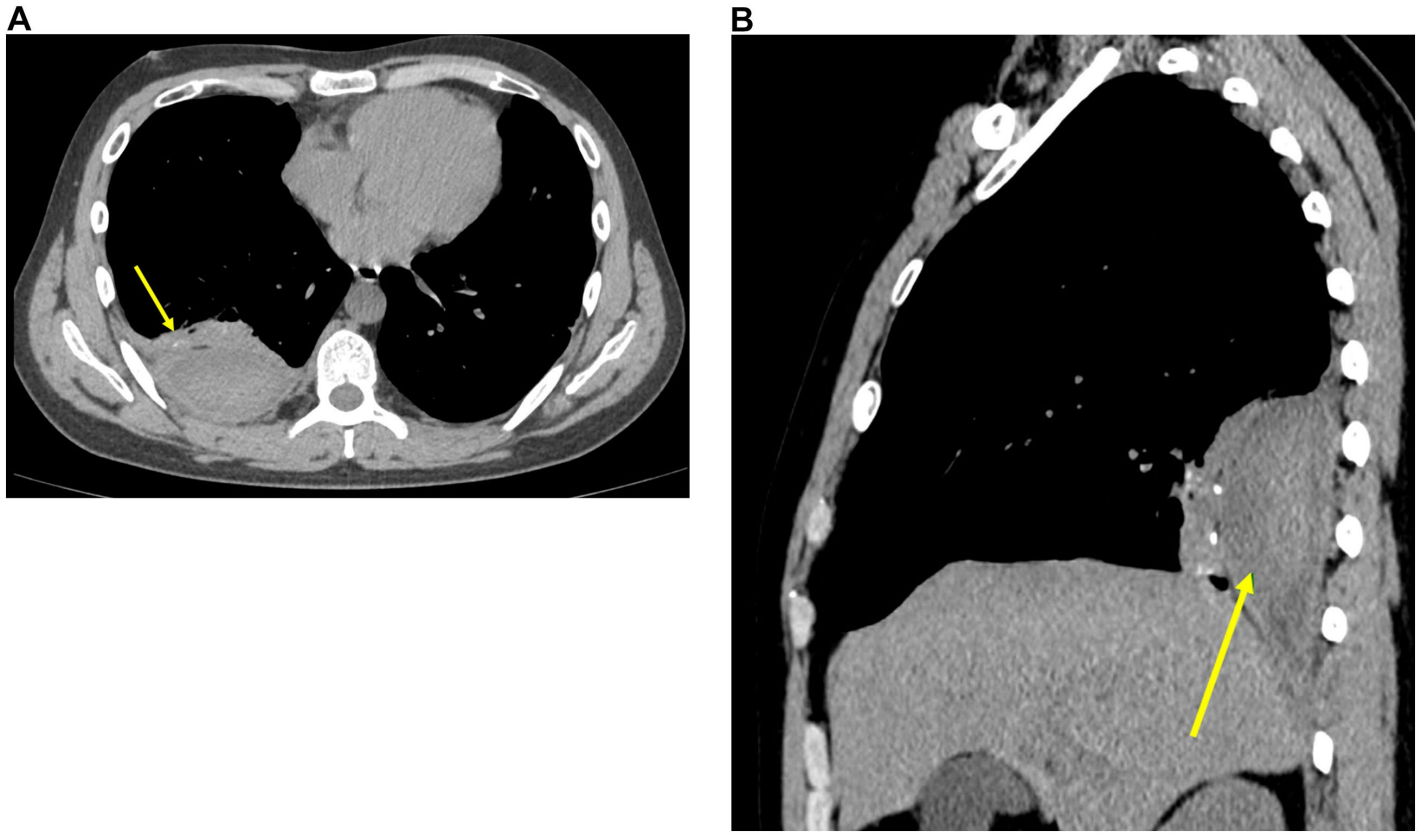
Follow-up chest CT (December 27, 2024). (A) Axial non-contrast CT shows a reduction in the size of the right extrapleural hematoma (arrow), with marked decrease in right pleural effusion and resolution of right lower lobe atelectasis. (B) Sagittal non-contrast CT demonstrating the residual right extrapleural hematoma (arrow).
